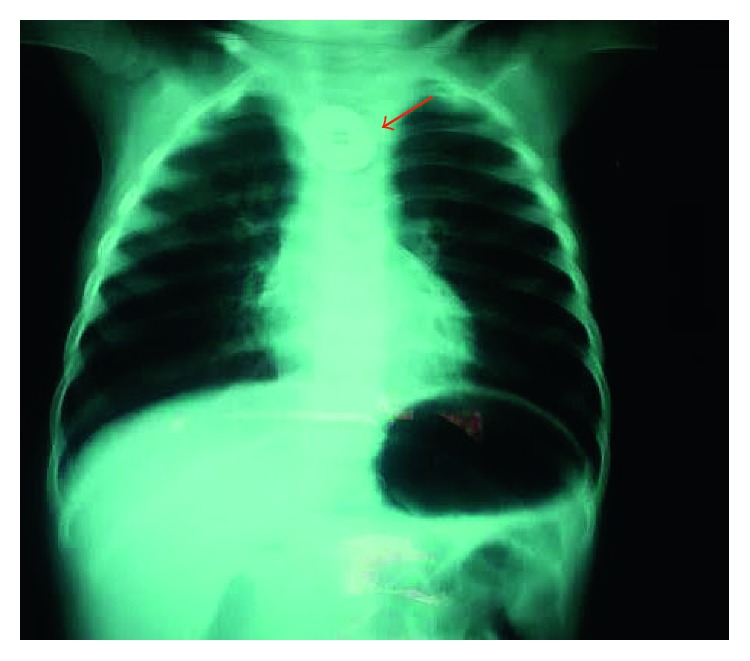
Anteroposterior chest X-ray showing a foreign body in the upper thoracic esophagus.
